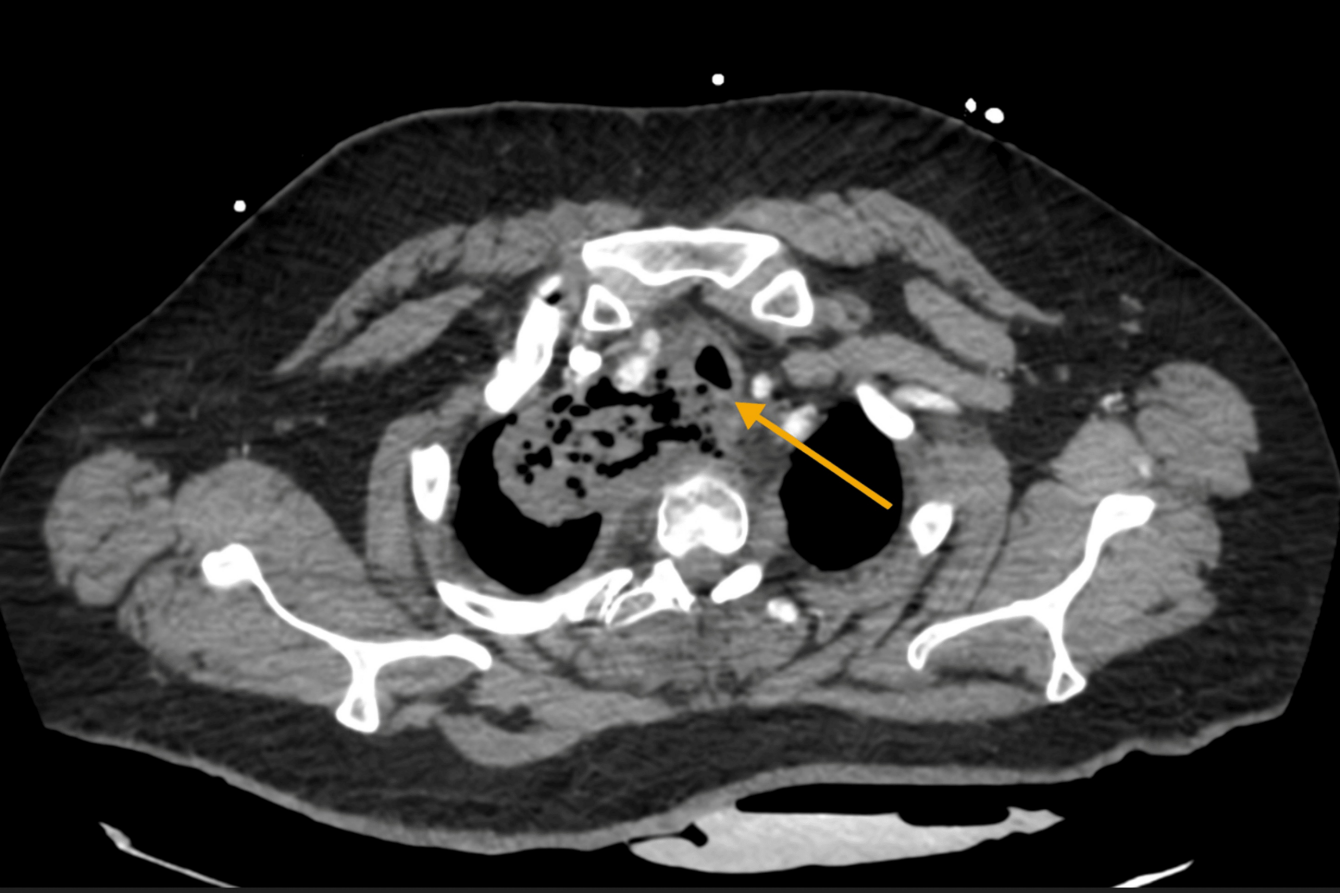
CT of the thorax showing esophageal dehiscence in the right paratracheal region, concerning for esophageal perforation
